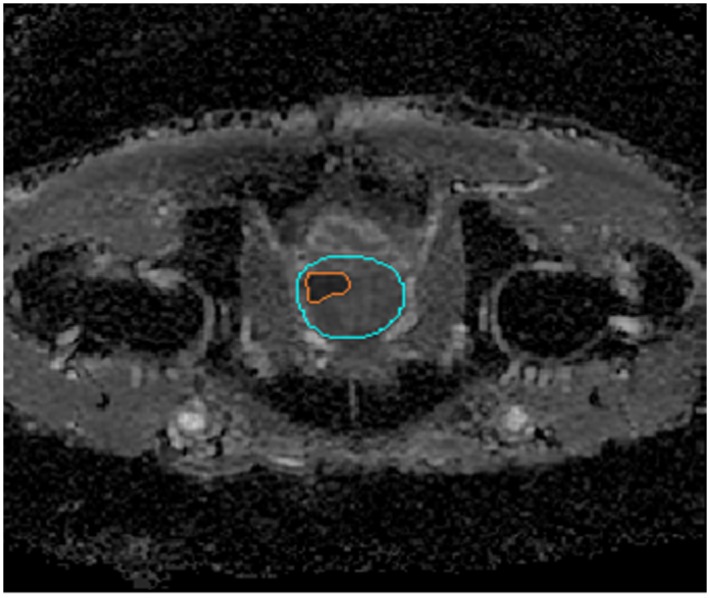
MR image of a tumour (in orange) and its surrounding tissue (in pale blue).
